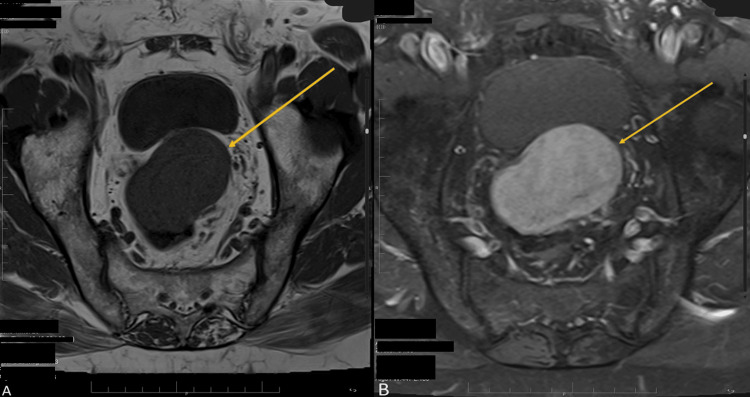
Abdominal MRI (T1-weighted images). (A) A T1 hypointense mural rectosigmoid mass. (B) A heterogeneous enhancement with areas of cystic changes on the post-contrast study.
